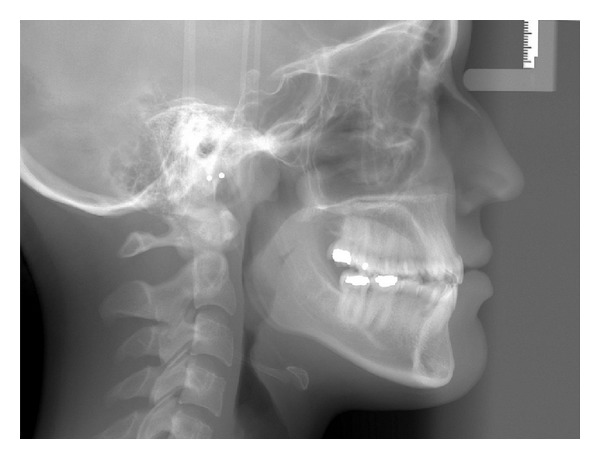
Initial lateral cephalometric radiograph.
